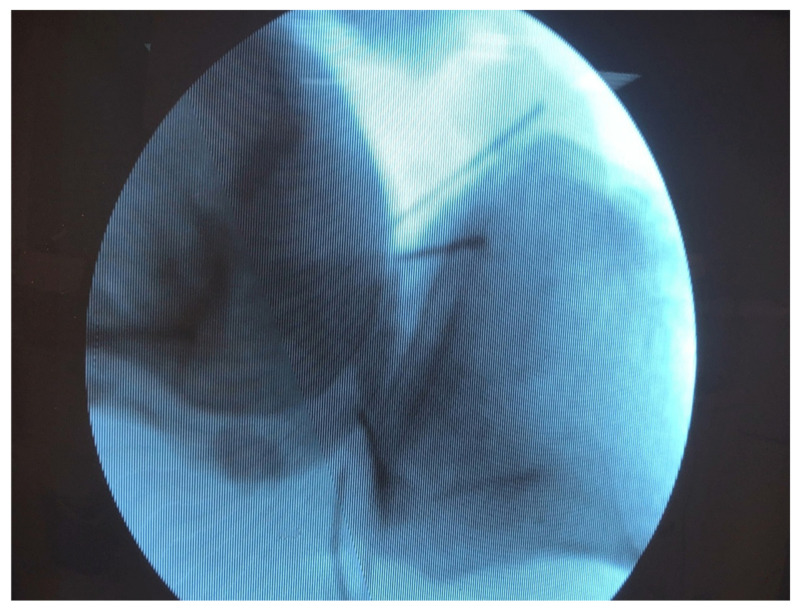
X-rays confirmed the proper placement of the pins.
